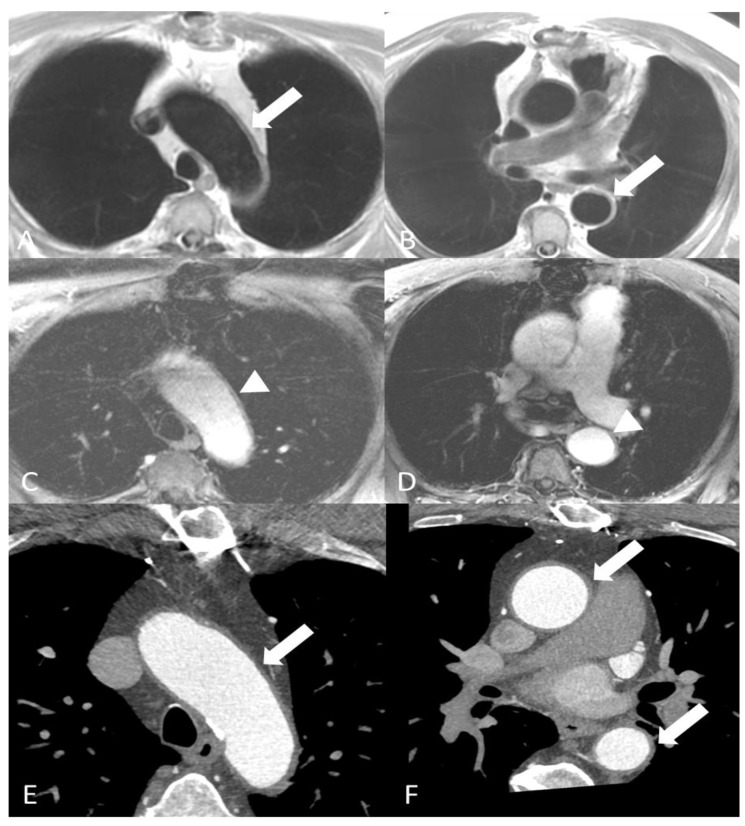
A 75-year-old man with known giant cell arteritis. Axial double inversion recovery MR images (A,B) demonstrating concentric mural thickening of the thoracic aorta (arrows). Axial T1-weighted images after intravenous contrast administration (C,D) demonstrating mild enhancement to the aortic wall more pronounced in the arch and descending aorta (arrowheads), consistent with active vasculitis. Images of chest CTA (E,F) showing concentric mural thickening of the ascending aorta, aortic arch and descending aorta (arrows).
